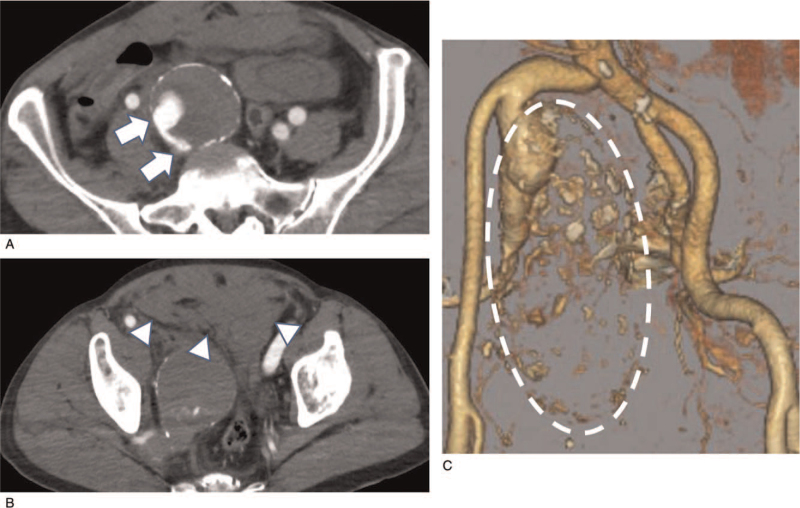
Preoperative CT. (A) CT reveals a giant IIIAA with a dimeter of 67 × 74 mm. Thick mural thrombus, and small volumes of contrast medium extravasation into the aneurysmal wall are observed (arrow). (B) Hematomas are found in the intraperitoneal and pelvic spaces (arrow head). (C) Three-dimensional CT shows a giant IIIAA (dotted line). CT = computed tomography, IIIAA = isolated internal iliac artery aneurysm.
